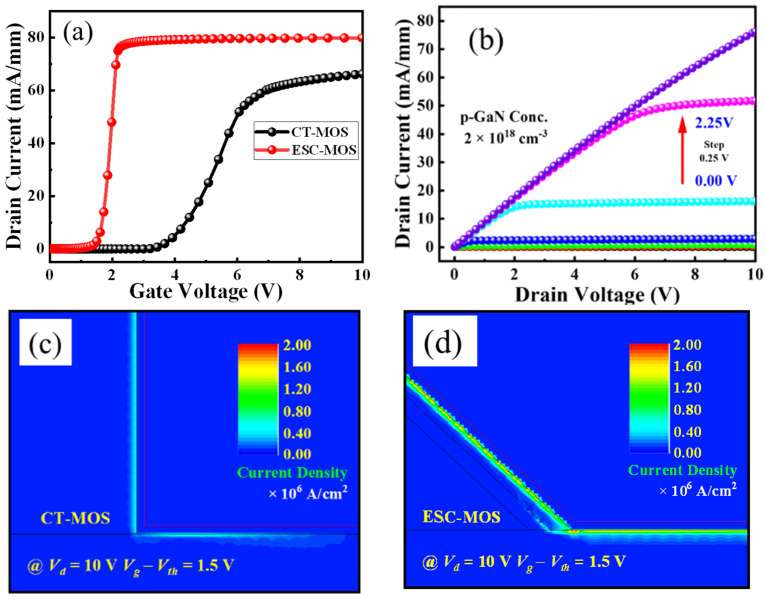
Transfer curves (a) of the CT-MOS and ESC-MOS devices. (b) is the typical output characteristic of ESC-MOS with 300 nm p-GaN (hole concentration of 2 × 10^18^ cm^−3^). (c,d) are the corresponding forward current distributions with an overdrive voltage of 1.5 V.
